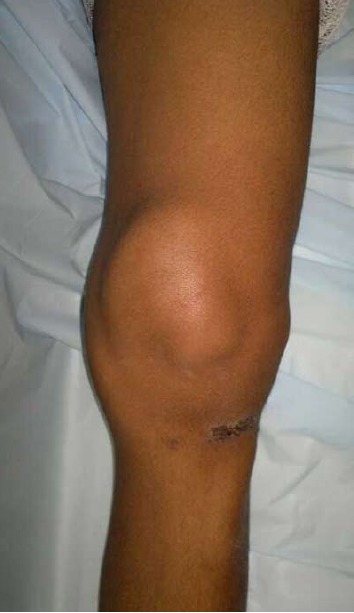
Fig. 2: MRI images showing the lesion between patella and femoral condyle. F2:
